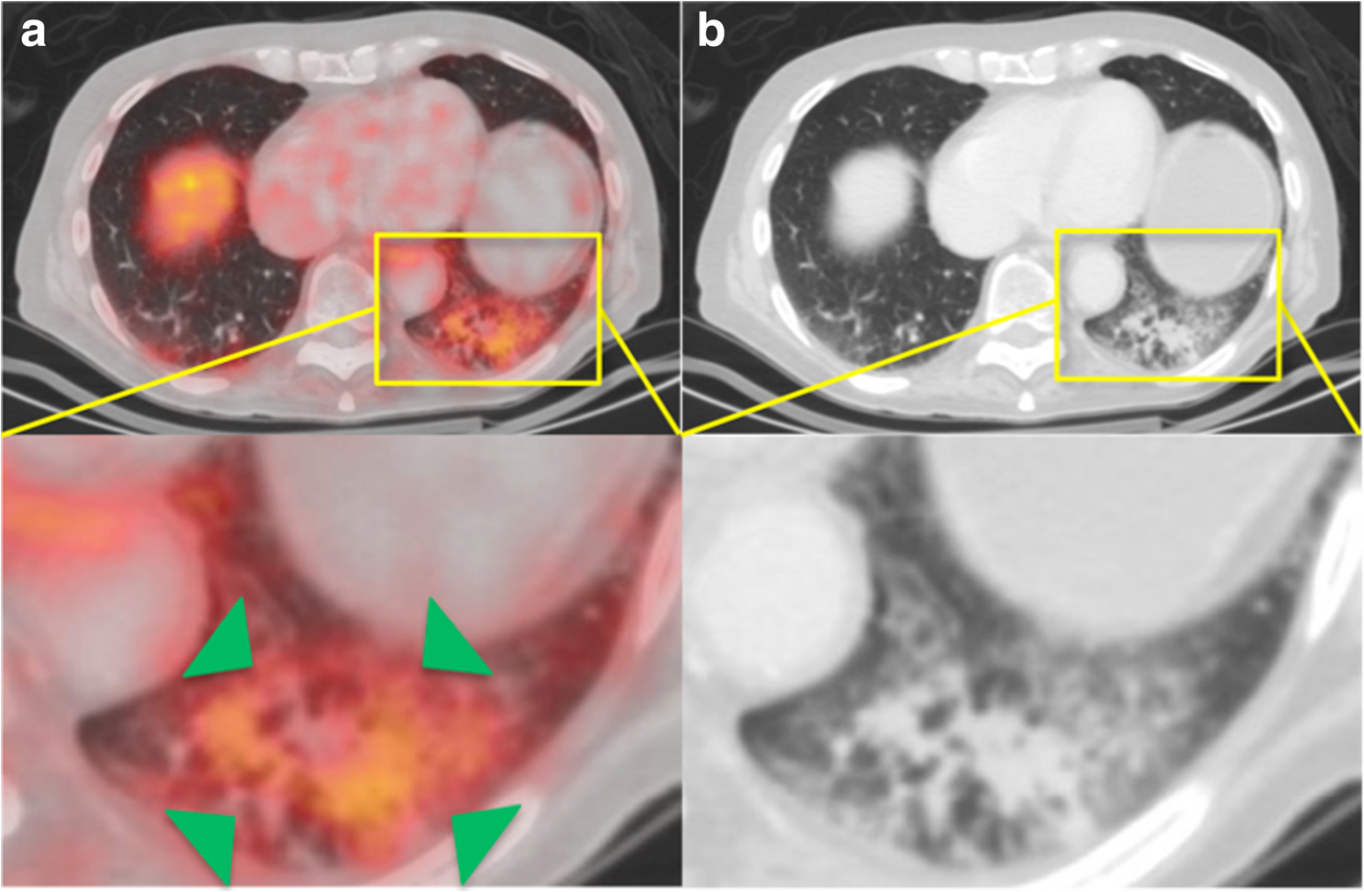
Example of pulmonary opacities in a PC patient. a, b: ^68^Ga-PSMA-PET/CT of an 82-year-old patient with an adenocarcinoma of the prostate. At initial diagnosis in 2010, the GS was 3+4. He had received androgen deprivation therapy only. The serum PSA was 6.48 ng/ml at the time of examination. PET/CT (a) revealed PSMA-positive peribronchial opacities, with SUV_max_-values up to 3.8 (green arrows). In CE-CT (b), the opacities appear as dense multifocal peribronchial opacifications and ground-glass-opacities, with partial confluence. It was interpreted as bronchopneumonia. *GS* Gleason score, *PSA* prostate-specific antigen, *SUV*_*max*_ Maximum standardized uptake value
